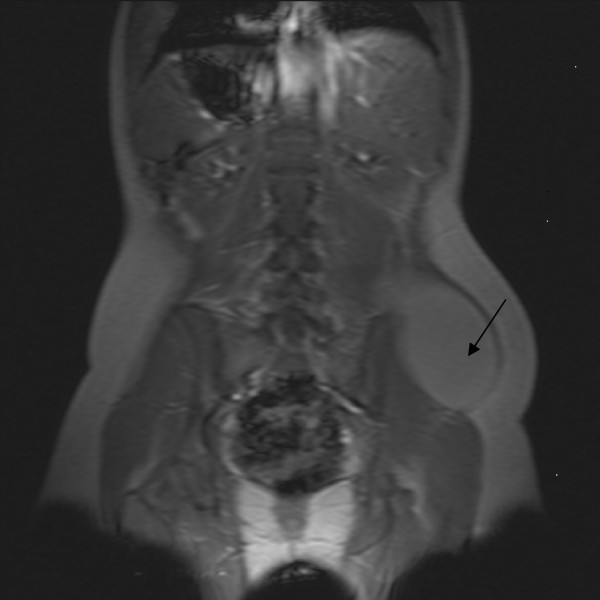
MRI scan in the coronal plane showing the lateral wall haematoma.
